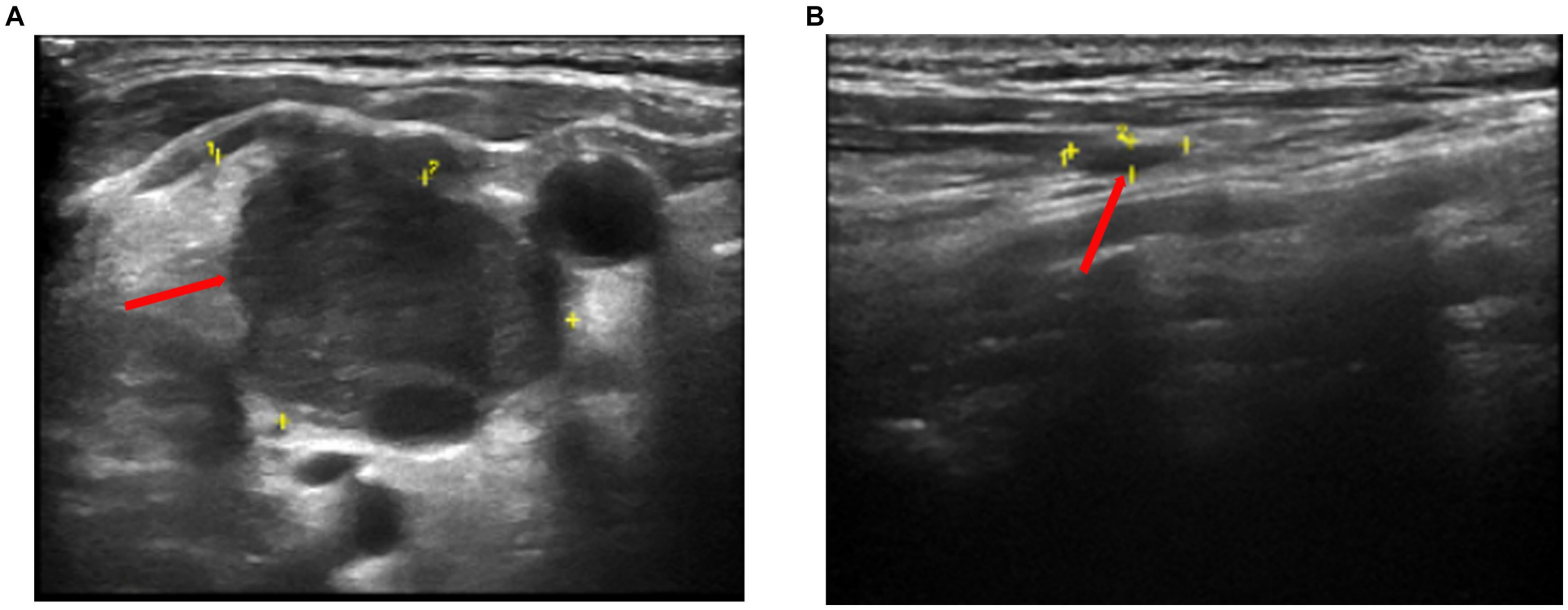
Color doppler sonography showed multiple echogenic lymph nodes of different sizes on both clavicles. (A) The larger one on the right is approximately 28 mm × 20 mm in size (yellow section and red arrow). (B) The larger one on the left is approximately 25 mm × 8 mm in size (yellow section and red arrow).
